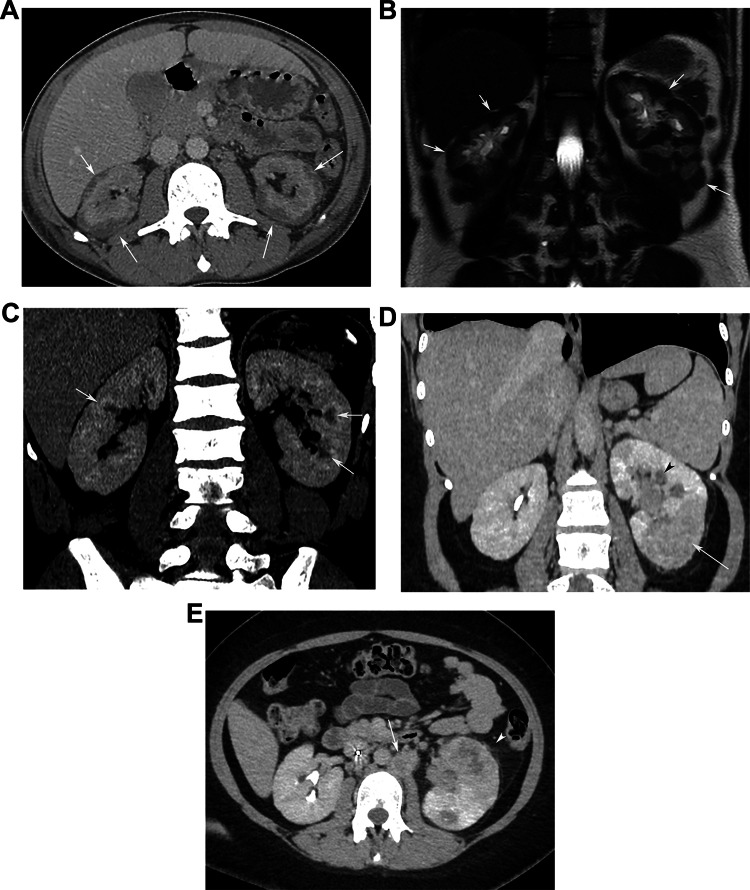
a–e Renal manifestations of sickle cell disease. a Post-contrast CT scan of a 34-year-old male with sickle cell disease, presenting with epigastric abdominal pain and elevated serum amylase and lipase levels, initially managed as severe pancreatitis. The clinical course was complicated by worsening hyperbilirubinemia and acute kidney injury requiring dialysis. The scan demonstrates bilateral renal cortical necrosis (arrows). b Coronal T2-weighted MRI of a 42-year-old male with long-standing sickle cell disease and multiple complications. The image demonstrates diffusely hypointense signal intensity in the renal cortex bilaterally (arrows), consistent with renal hemosiderosis. The liver and spleen also show diffuse low signal intensity on T2-weighted imaging, indicative of iron deposition. c Equilibrium-phase post-contrast CT scan of a 30-year-old male presenting with intense pain involving both upper and lower extremities, as well as the lumbar region. Past medical history includes avascular necrosis of the femoral head. The image shows bilateral triangular-shaped areas with reduced contrast enhancement in the renal medulla, consistent with papillary necrosis. d, e Equilibrium-phase post-contrast CT scan of a 26-year-old female with sickle cell trait, presenting to the emergency department with abdominal pain. The scan demonstrates an infiltrative hypovascular renal mass (arrow in d) associated with collecting system dilatation (arrowhead in d), perinephric infiltration (arrowhead in e), and lymphadenopathy (arrow in e)
